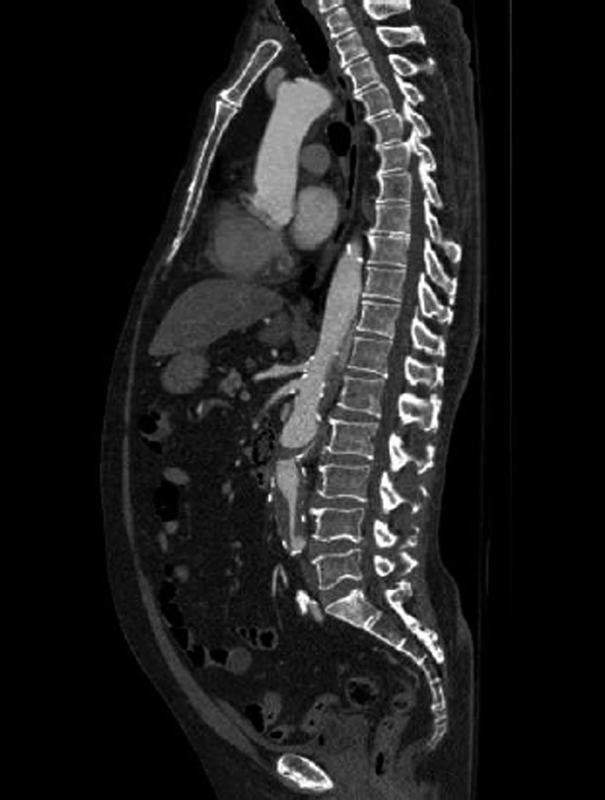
Computed tomography angiography showing the subtotal occlusion of the aortic lumen just below the level of the renal arteries.
